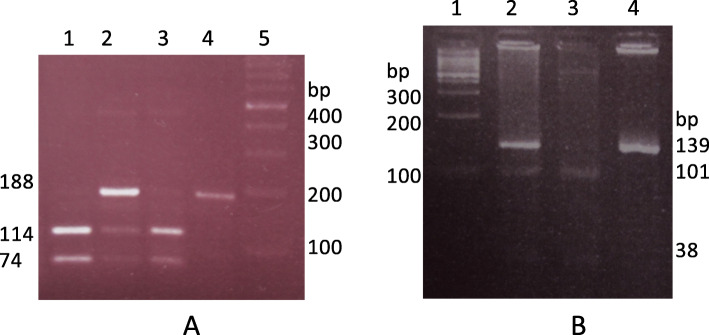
3% agarose gel electrophoresis of the restriction enzyme digestion (a) 188 bp PCR fragment of the *MUC1* gene restriction enzyme digestion results using *AIWNI* enzyme; lane 1 and 3 shows homozygous AA, lane 2 heterozygous GA genotype and lane 4 shows homozygous GG genotype respectively, lane 5 shows 100 bp DNA marker (b) Restriction enzyme map of the 139 bp PCR fragment of the *PSCA* gene using *NIaIII* enzyme. Lane 1 = 100 bp DNA marker, lane 2 heterozygous CT, lane 3 (101 and 39 bp) shows homozygous TT and lane 4, uncut PCR product shows homozygous CC genotype respectively
